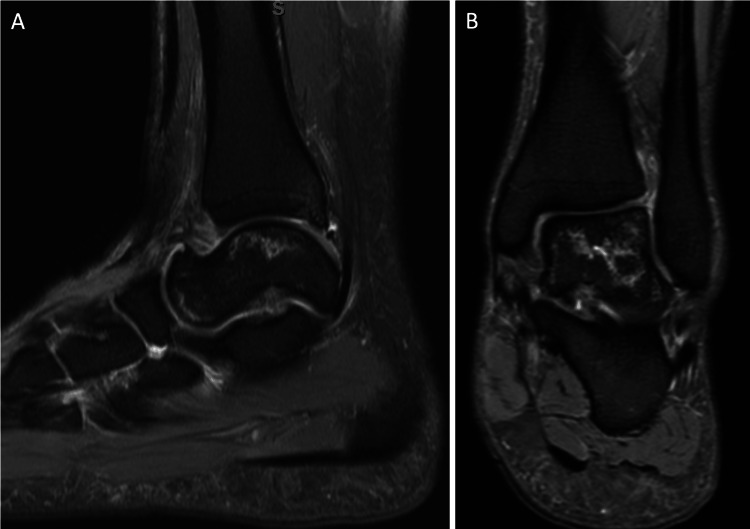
MRI of the left ankle at 15 months from injury - January 31, 2022. (A-B) T2-sequence, sagittal and coronal views show signs of osteonecrosis resolution with no collapse. The posterior tibial plafond re-demonstrates a stable small osteochondral defect.
